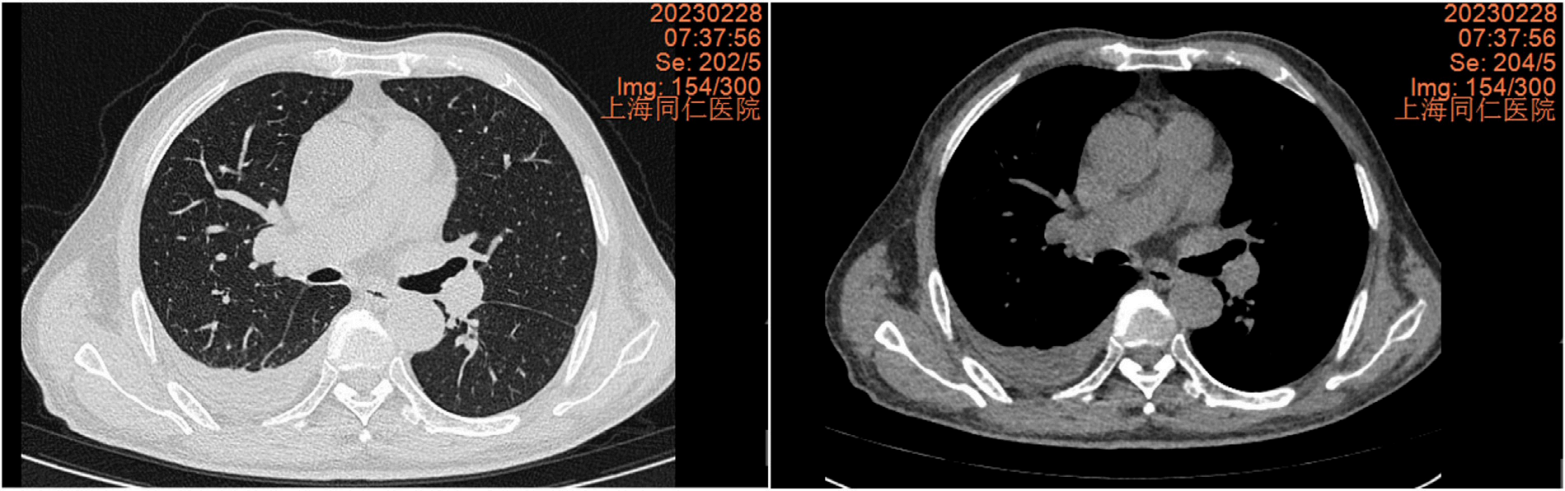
Chest CT scan from 28 February 2023, showing right pleural effusion and incomplete right lower lung atelectasis.
